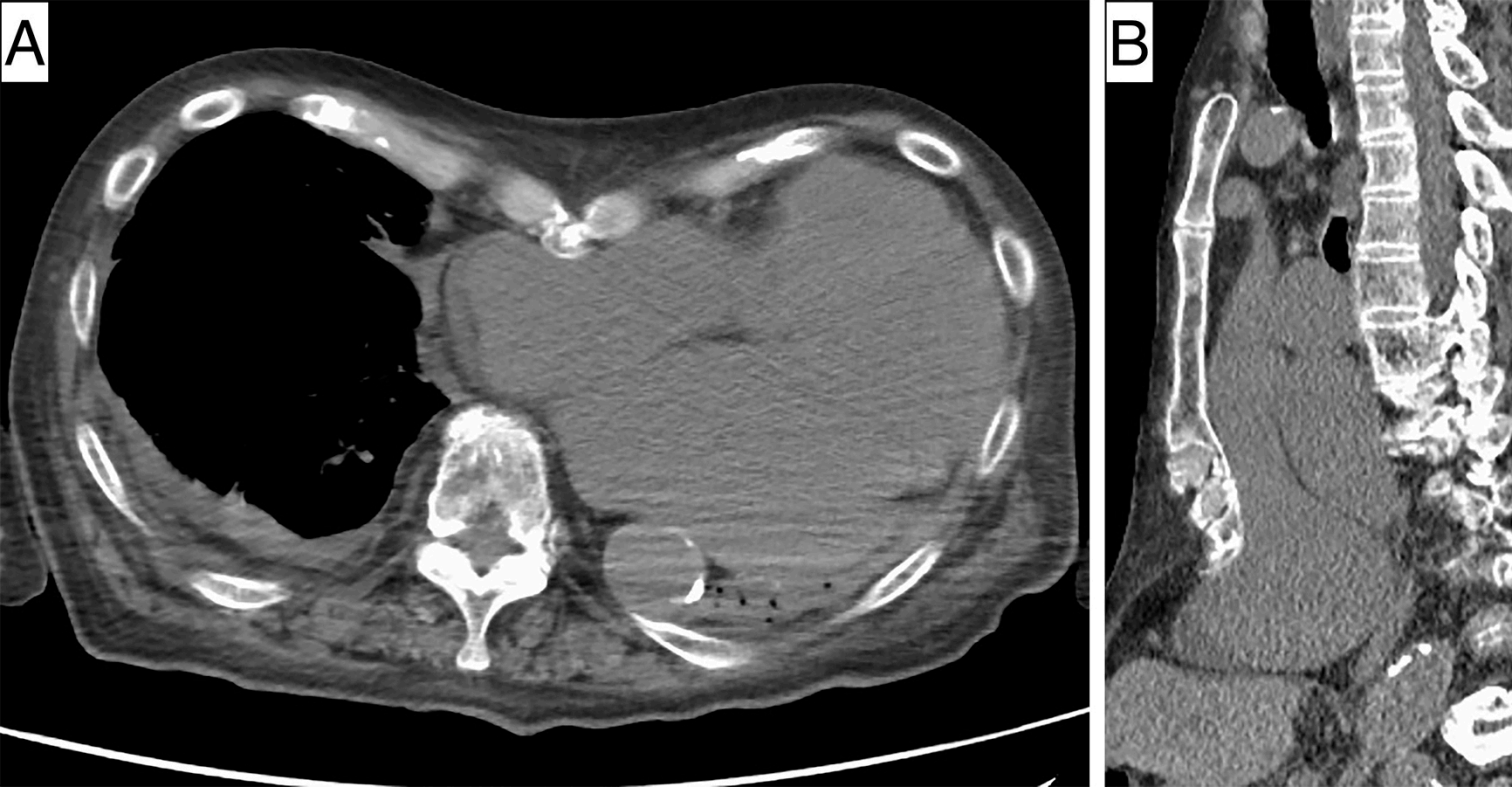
Chest computed tomography showing that the patient’s enlarged heart was pushed leftward by the narrowed chest cavity caused by the pectus excavatum, and his heart “wedged” into the left cavity, causing almost complete occupancy of the left thoracic cavity by the heart and a disturbance in left lung expansion.
